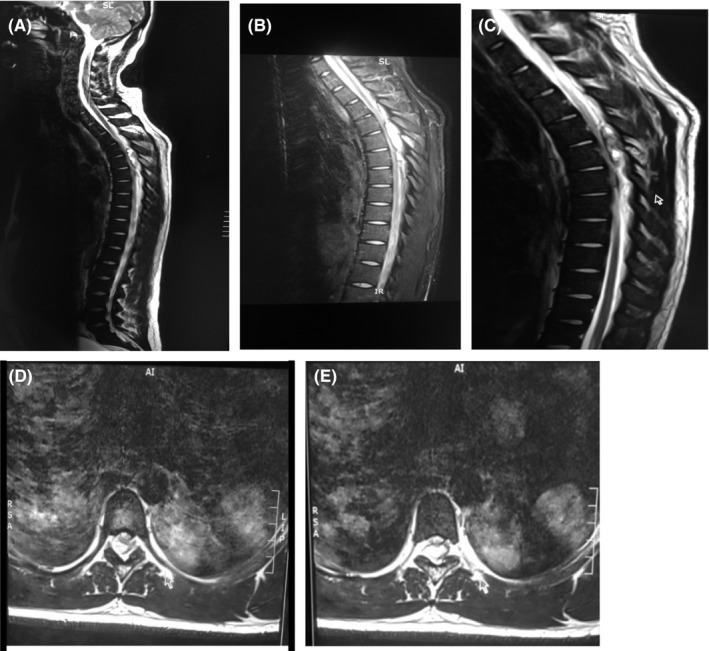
MRI spine sagittal (A, B, C) and axial (D, E) view showing a hyperintense macrolobulated epidural mass with possible intralesional bleed causing spinal cord compression from midvertebral body of T3 to T6
